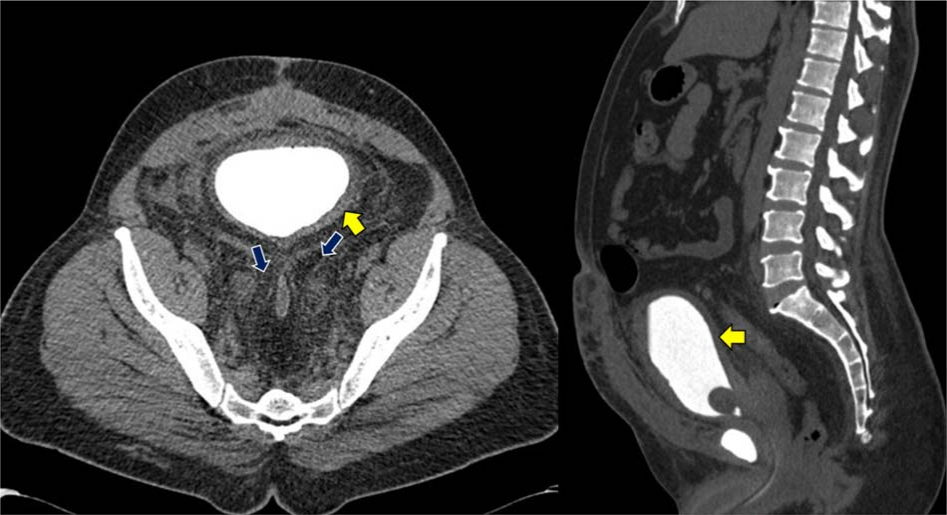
Axial and sagittal CT cystogram demonstrating urinary bladder wall thickening (yellow arrow) with PL and extensive fat strandings (blue arrows).
